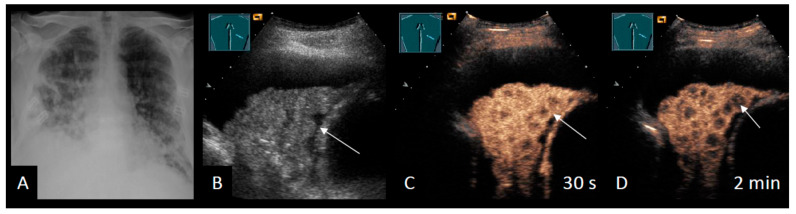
Patient with MPE secondary to renal cell carcinoma. (A) A chest X-ray shows multiple, diffuse opacities and a right-sided pleural effusion. (B) B-mode US displays an inhomogeneous atelectasis with an irregular surface (arrow). (C) CEUS shows an inhomogeneous enhancement with several hypoenhancing intrapulmonary lesions. (D) These lesions reveal a marked washout after two minutes, indicating diffuse lung metastases.
